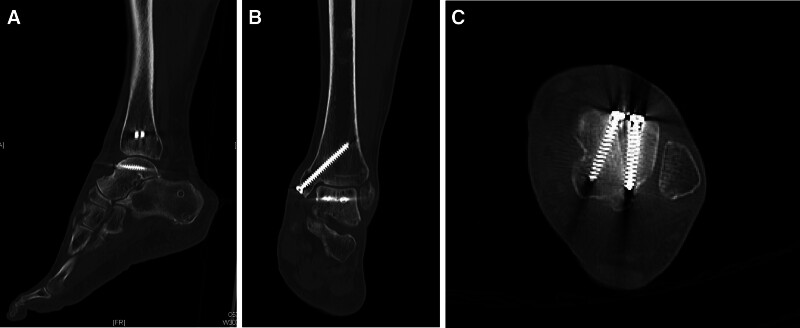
Follow-up CT with three-dimensional reconstruction of left foot obtained 10 months after surgery, revealing fracture site bony union and no post-traumatic osteoarthritis.
